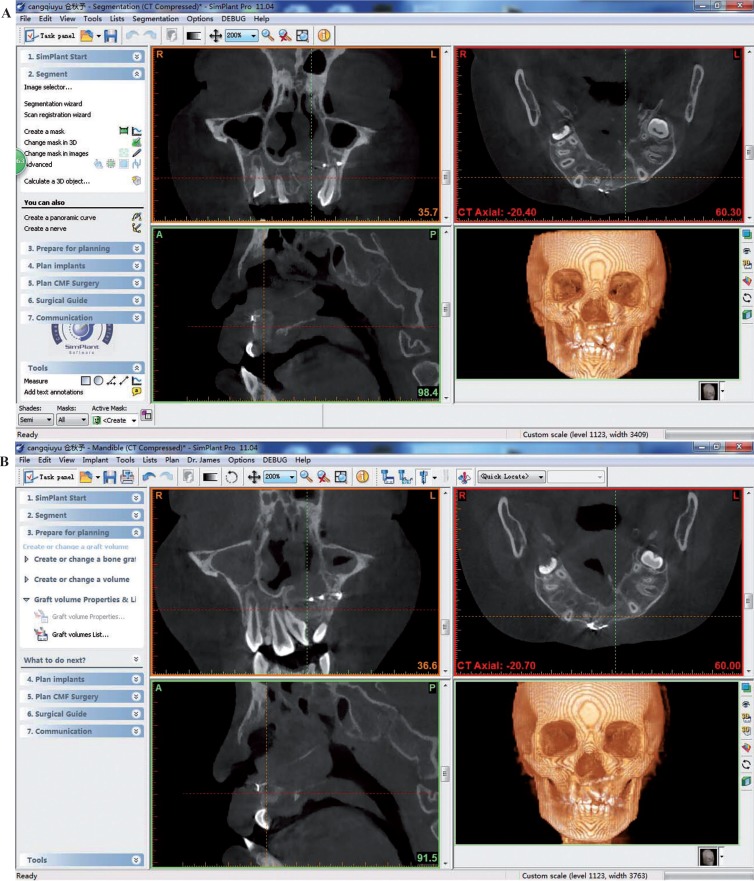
CBCT at 3- and 6-month postoperatively showed new bone formation in the bone graft site. A) The CBCT evaluation at 3-month postoperatively. B) The CBCT evaluation at 6-month postoperatively.
